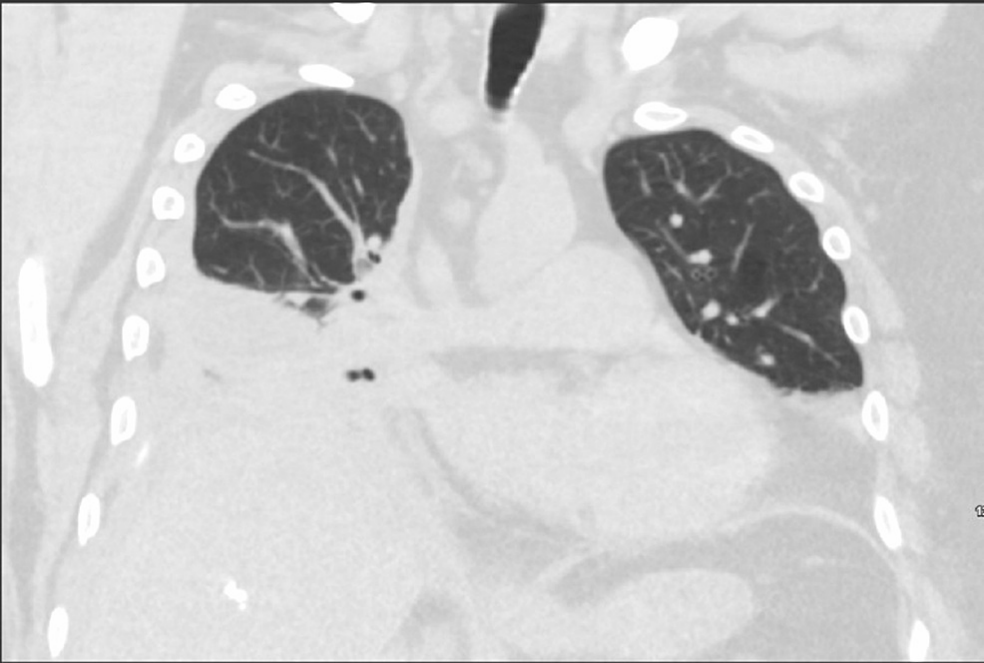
CT chest demonstrating right-sided pleural effusion
